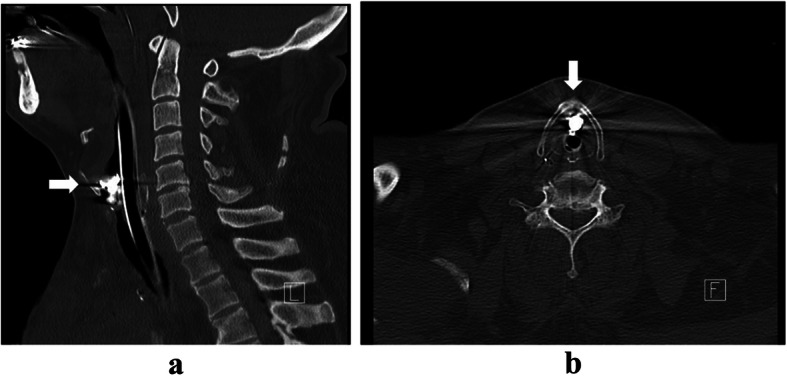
Simple CT scans on POD 34. a Sagittal section. An airway foreign body (arrow) was found on the ventral side of the tracheal tube at the level of the 5th and 6th cervical vertebrae. b Horizontal section. An airway foreign body (arrow) with metal artifacts was observed. CT, computed tomography; POD, postoperative day
